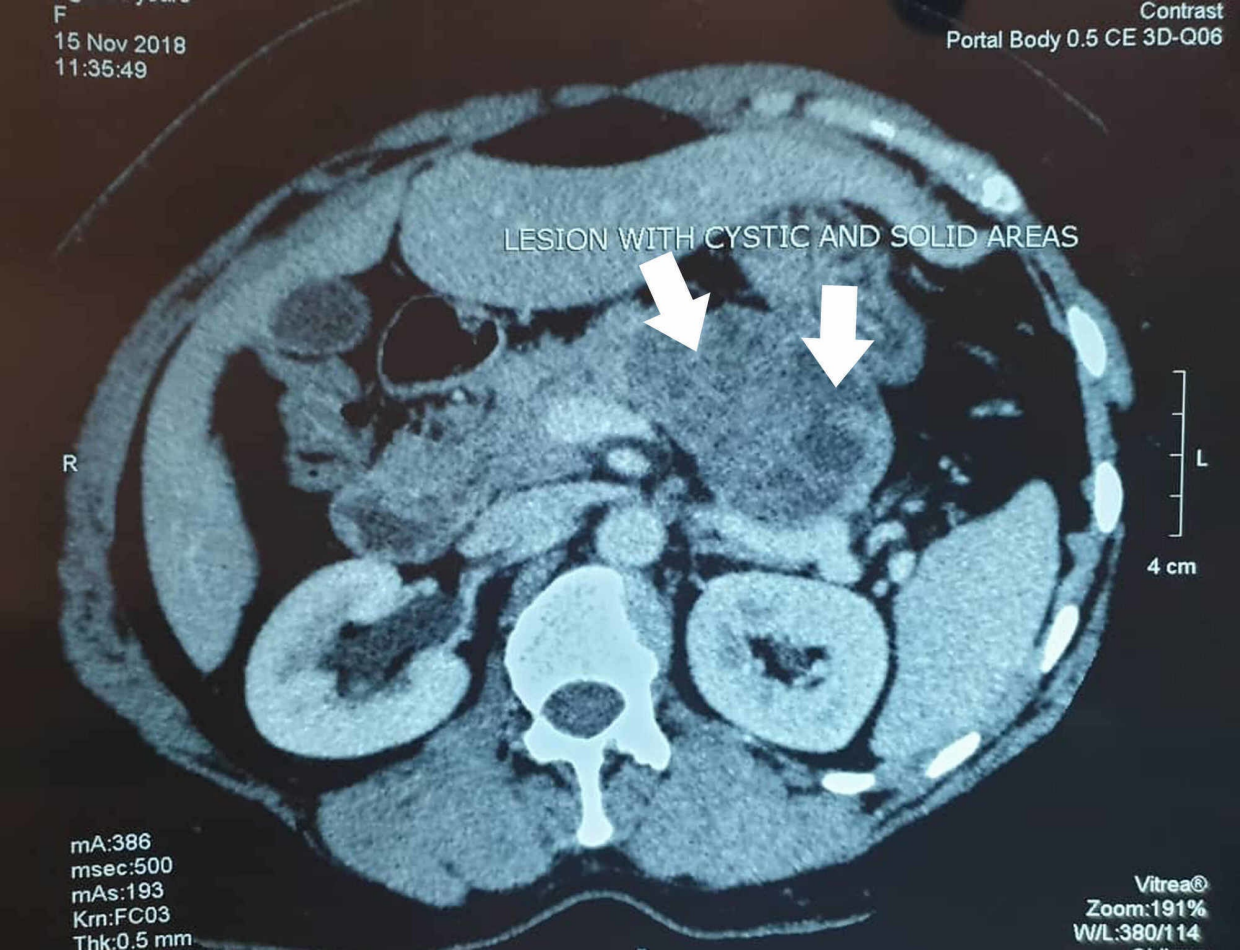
CECT showing the mass in the body and tail of the pancreas with solid areas alternating with cystic spaces (white arrows) CECT, contrast-enhanced computed tomography
